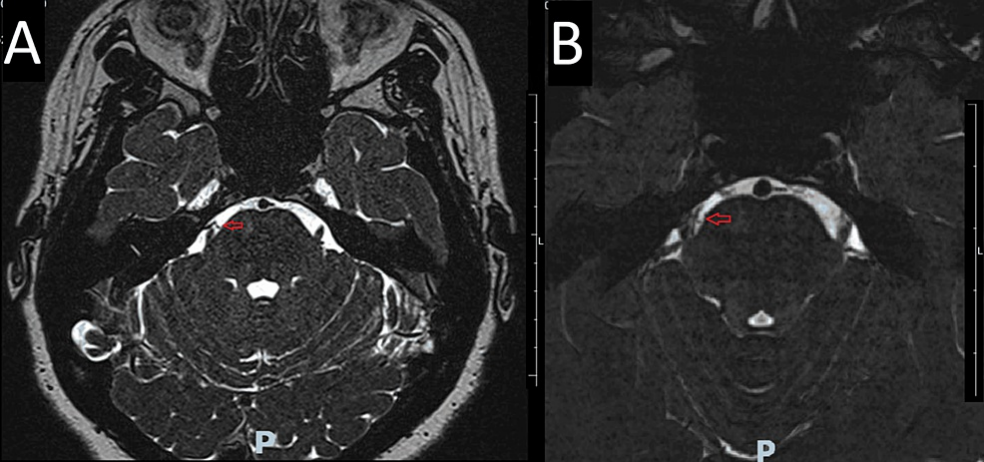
Axial magnetic resonance imaging (MRI) Axial view of an MRI T2 weighted image showing vascular conflict between the right trigeminal nerve and superior cerebelar artery in the entry zone (red arrows 1A and 1B).
